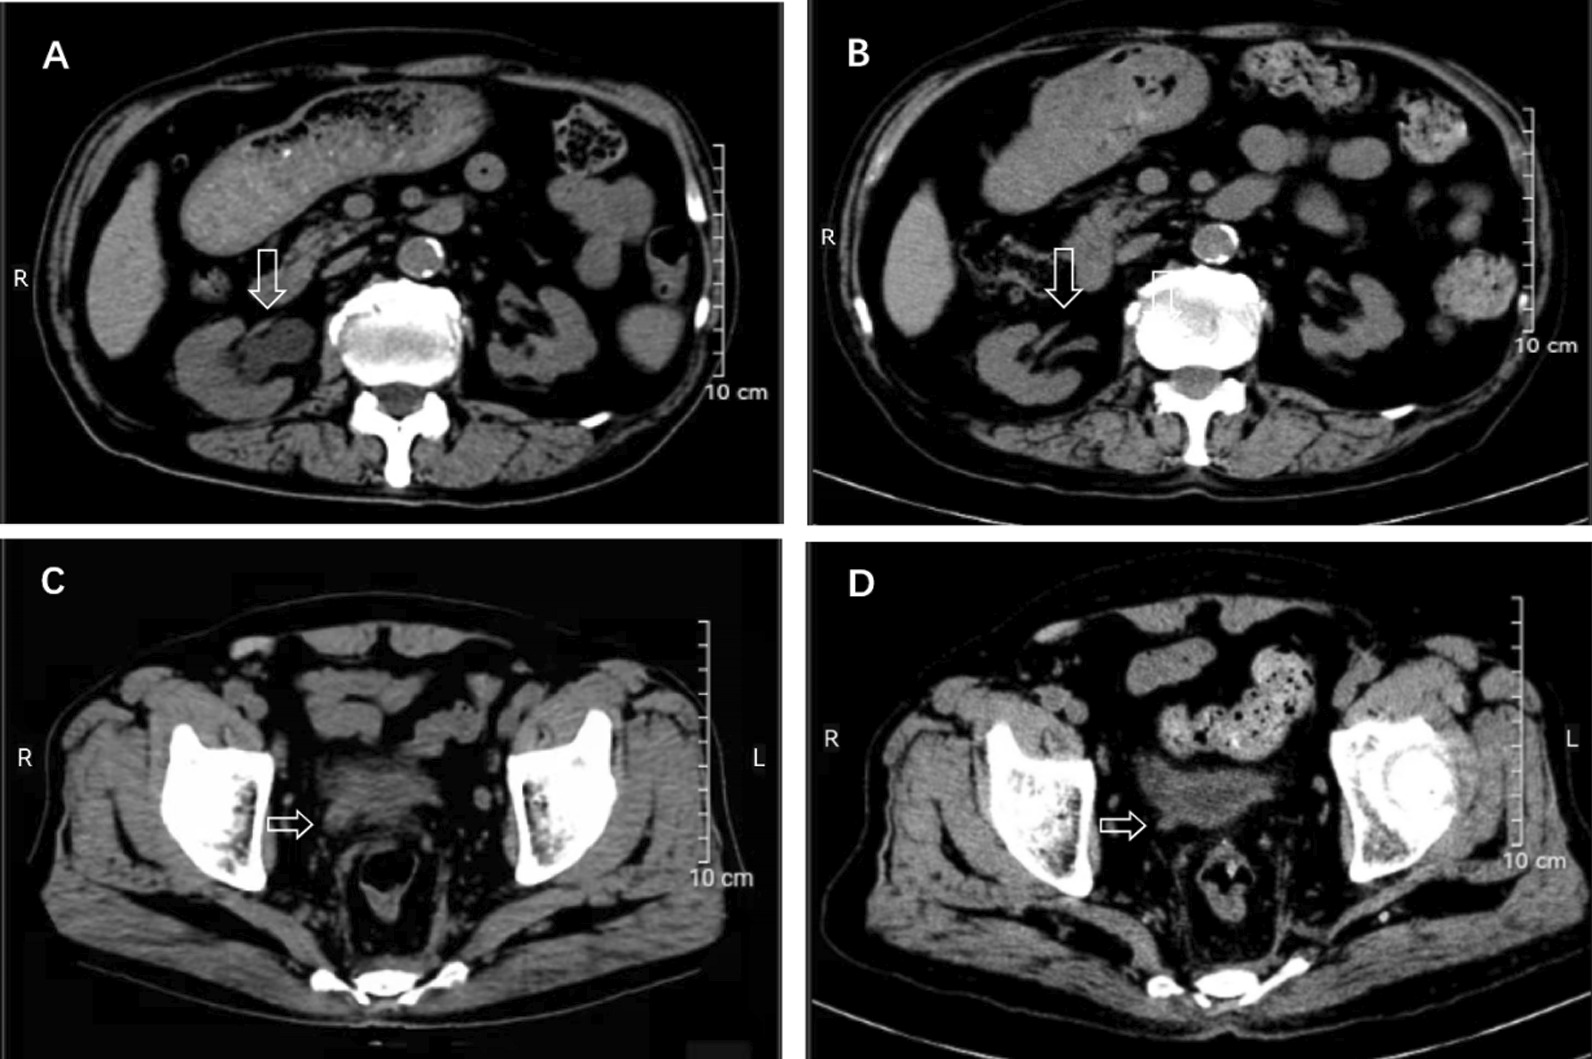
A CT image of the urinary system in December 2020, showing the right renal and ureter hydronephrosis. B CT image of the urinary system in December 2021 showed that the right renal and ureter hydronephrosis had disappeared. C CT image of the urinary system in December 2020 showed patchy soft tissue-like density shadows at the bladder inlet at the lower end of the right ureter. D CT image of the urinary system in December 2021 showed that patchy soft tissue-like density shadows at the bladder inlet had disappeared
